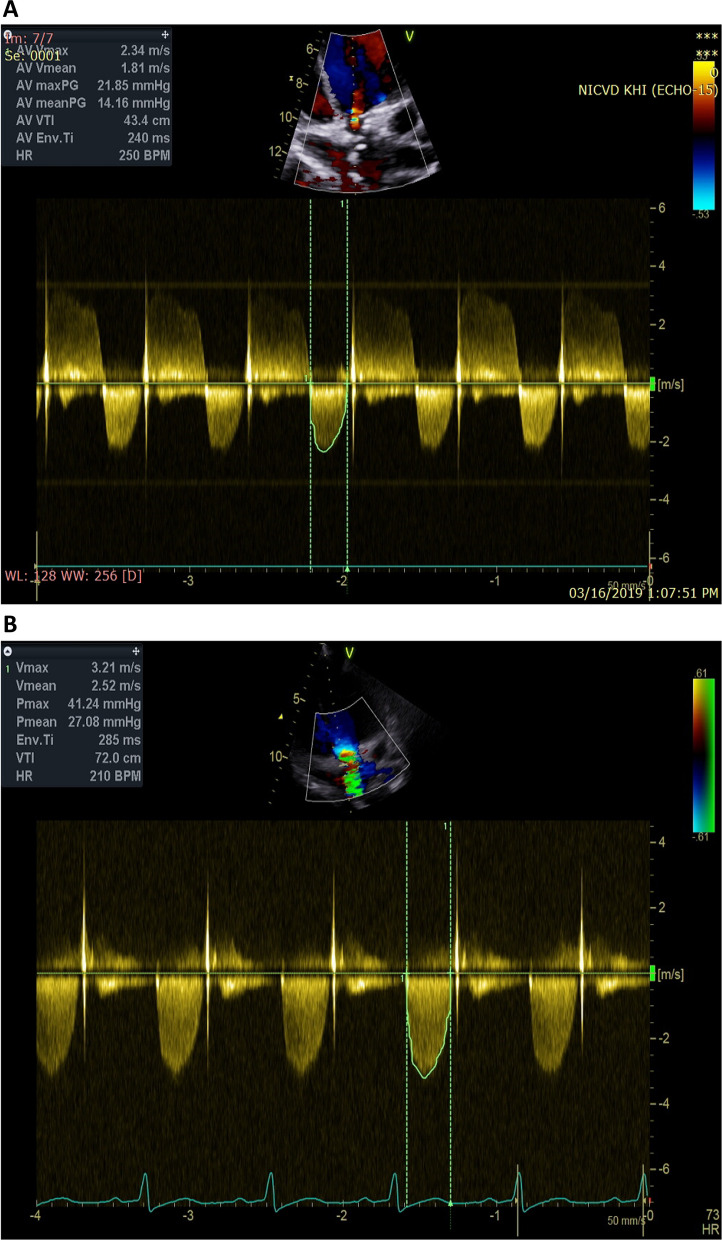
a Continuous wave Doppler on TTE showing gradient across Aortic valve, b which increases after coronary revascularization after increasing in LV systolic function
